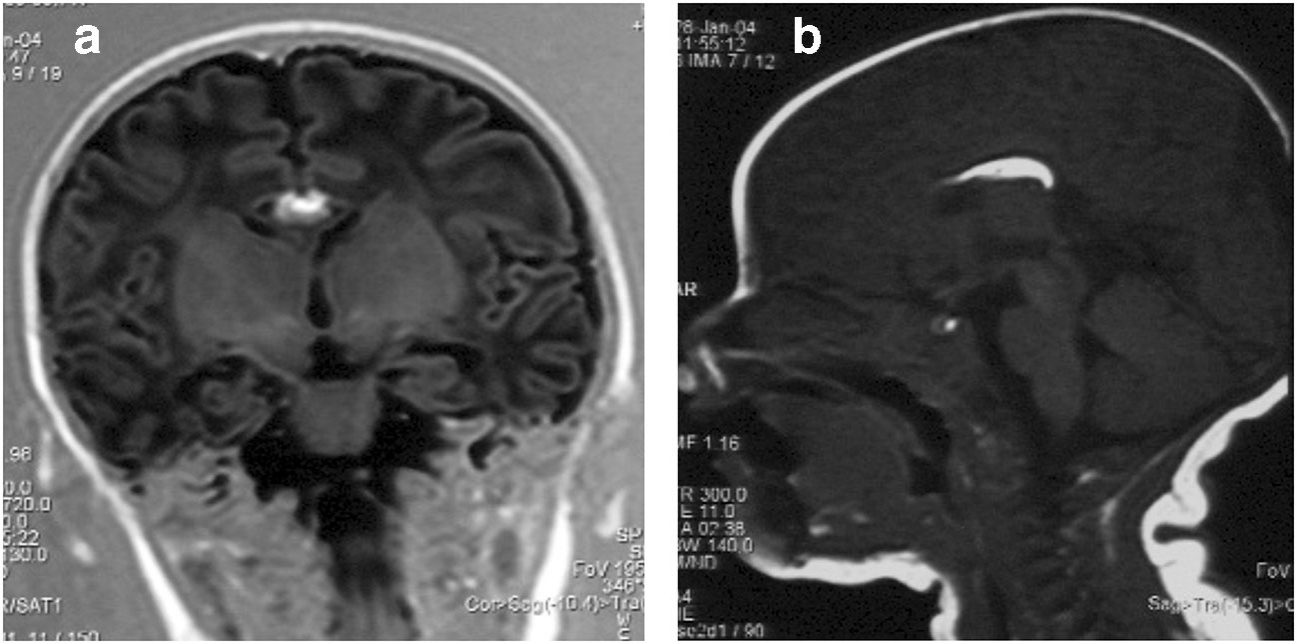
CORONAL and SAGITTAL views of brain MRI: lipoma as a thick hyper-intense band and partial agenesis of the corpus callosum [7]
